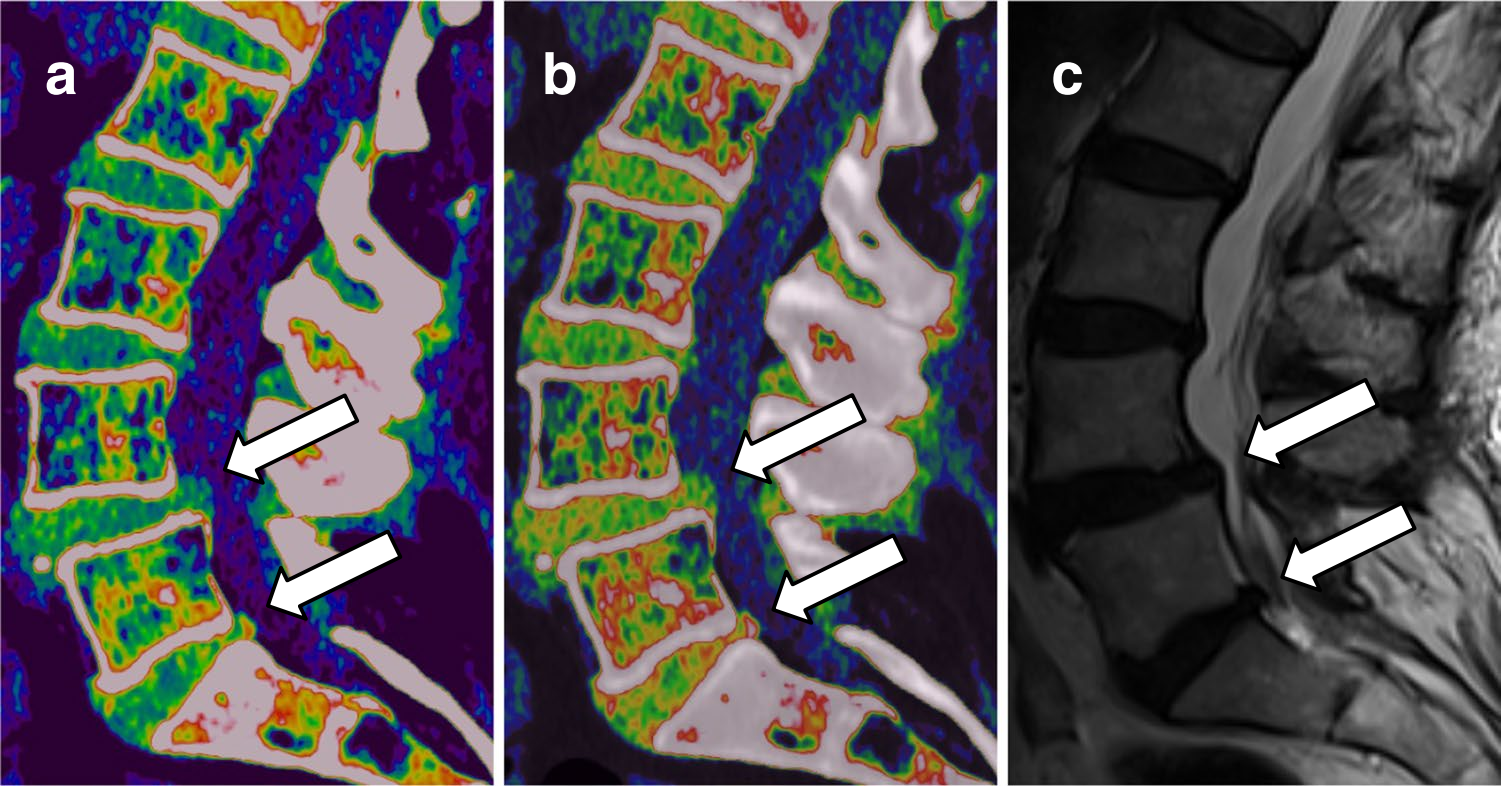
Case of a 61-year-old man with malignant melanoma who presented to the emergency department with severe lower back pain after lifting heavy weights at work. The patient initially underwent spine dual-energy CT imaging and afterwards MRI due to persistent lower back pain. Unenhanced sagittal VNCa reconstruction (a) depicted median lumbar disk protrusions at levels L4/5 and L5/S1 (*arrows*) and bulging of L3/4. Both, lumbar disk protrusions and bulging, were also clearly visible on sagittal VNCa CT series derived from contrast-enhanced portal venous phase (*arrows*) (b). T2-weighted MRI series confirmed the diagnosis of median lumbar disk protrusions at level L4/5 and L5/S1, as well as bulging of L3/4 on the sagittal plane (*arrows*) (c)
